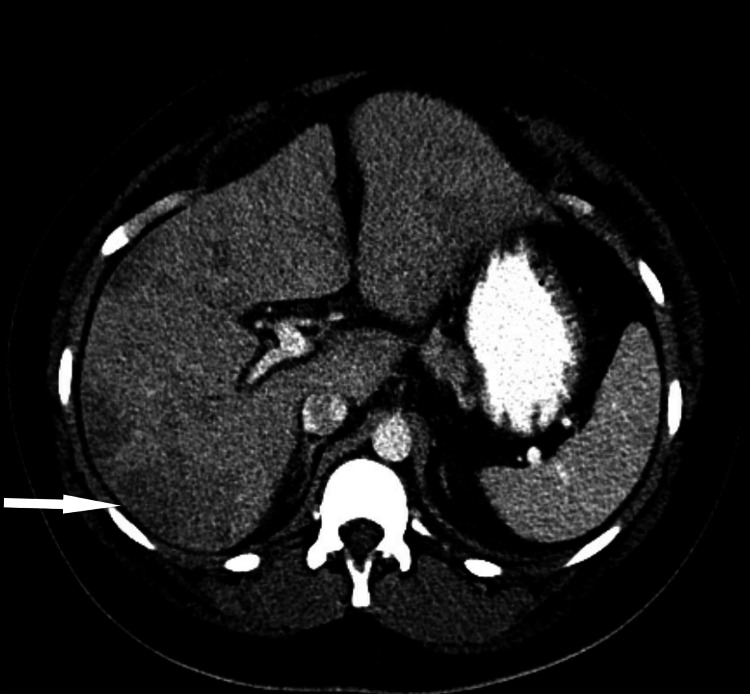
Contrast CT of the abdomen showing areas of hepatic infarction. CT: computed tomography
